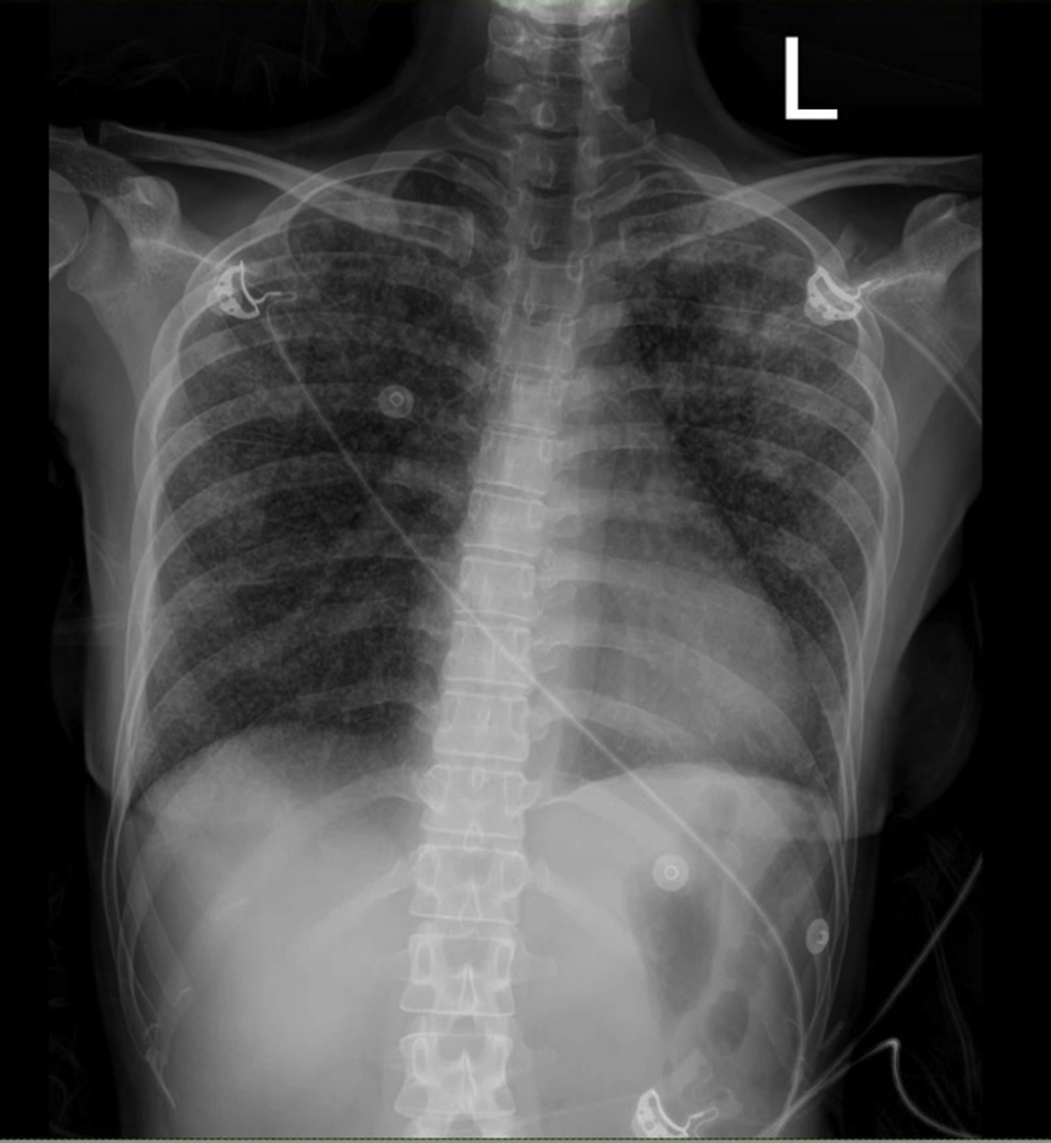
Chest Radiography Showing Diffuse Miliary Changes of the Lungs
